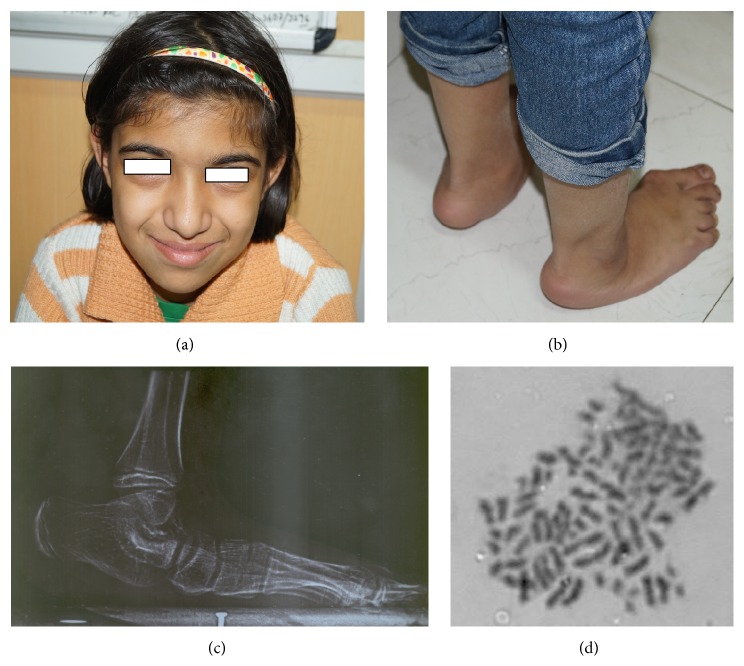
Mosaic triploidy case showing facial profile (a), flat rocker bottom foot ((b) clinical and (c) X-ray), and triploid metaphase cell (d).
